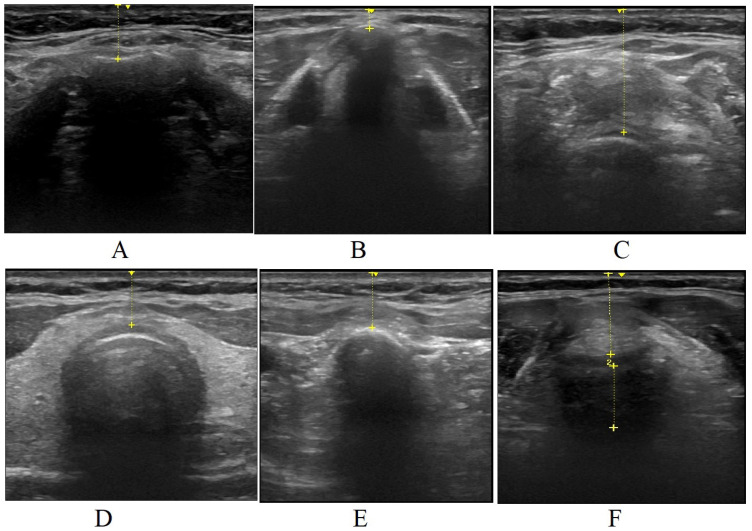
ANS thickness levels. A) ANS thickness at hyoid bone level. B) ANS thickness at vocal cord level. C) Preepiglottic ANS thickness at thyrohyoid membrane level. D) ANS thickness at isthmus level. E) ANS thickness at suprasternal notch level. F) Preepiglottic distance to distance between the epiglottis and the midpoint of the vocal cord. Sonographic measurement of PE/E-VC ratio.
